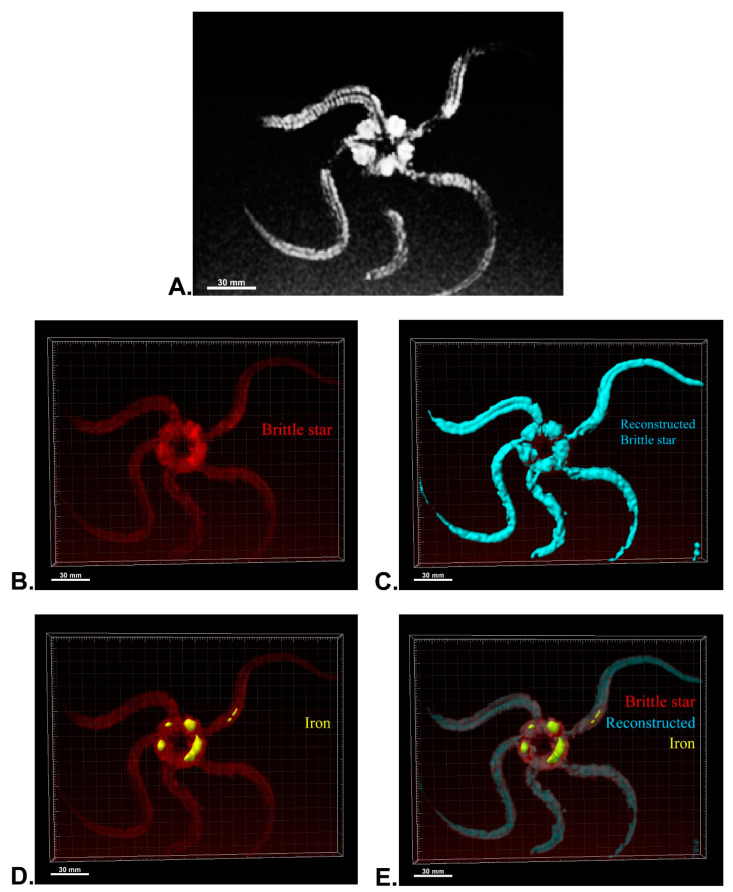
Three-dimensional (3D) reconstruction of brittle star tissue treated with iron using magnetic resonance imaging (MRI) serial images: (A) original grayscale image; (B) whole tissue imaging and signals of the brittle star tissue without reconstruction; (C) brittle star whole tissue 3D reconstruction in which the blue surface represents reconstructed brittle star tissue; (D) 3D reconstruction of the iron signals (shown in yellow); (E) 3D reconstruction of the whole tissue of brittle star with its all signals. All scale bars in four images are 30 mm. All images were processed and generated by Imaris software (V 7.4.2, ImarisX64; Bitplane AG).
